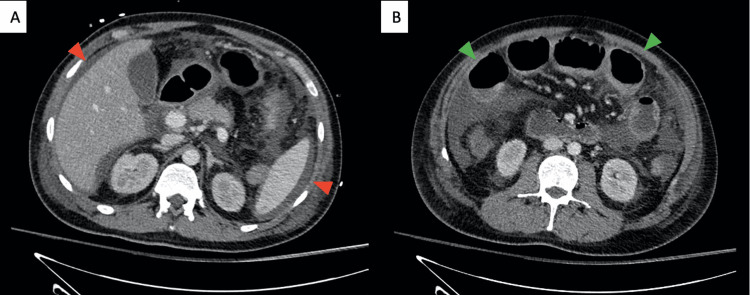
Contrast-enhanced computed tomography in the portal phase the day after admission Two CT images showing axial scans through the upper third (A) and middle third (B) of the abdomen. It shows multi-compartmental ascites (red arrows), peritoneal enhancement, dilated small intestine loops (green arrows), and no evidence of gastrointestinal perforation. CT: computed tomography
